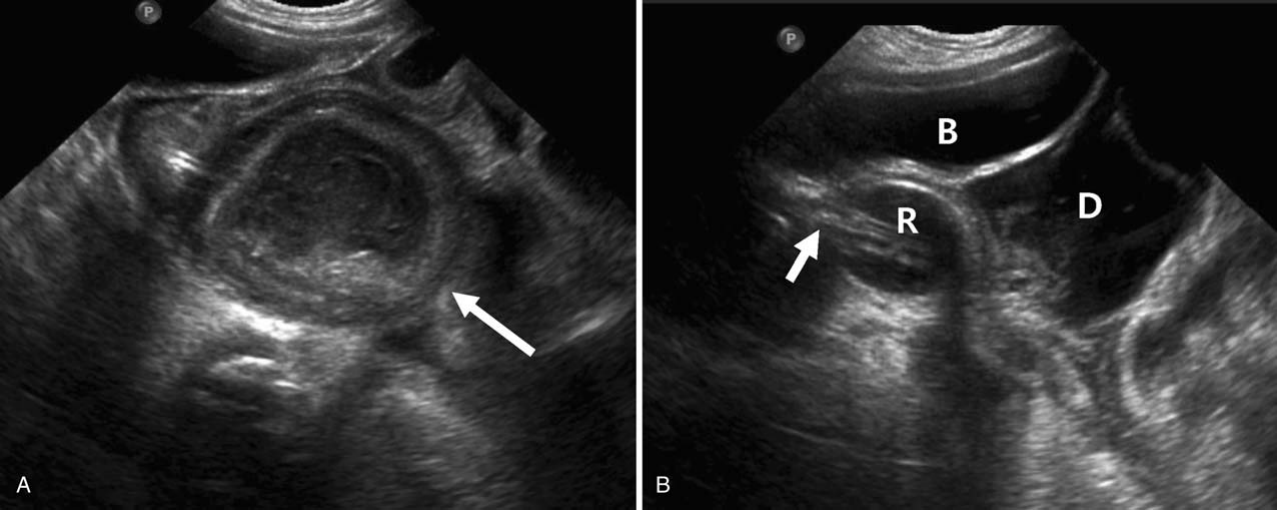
(A) Ultrasound showed bowel wall thickening with extremely distended colon and rectum, which indicated toxic megacolon. (B) Ultrasound after foley insertion through the anus. The balloon tip of the foley catheter passed through the true rectum adjacent to the distended duplication bowel. (Arrow: foley catheter, B: bladder, D: duplication cyst, R: rectum).
